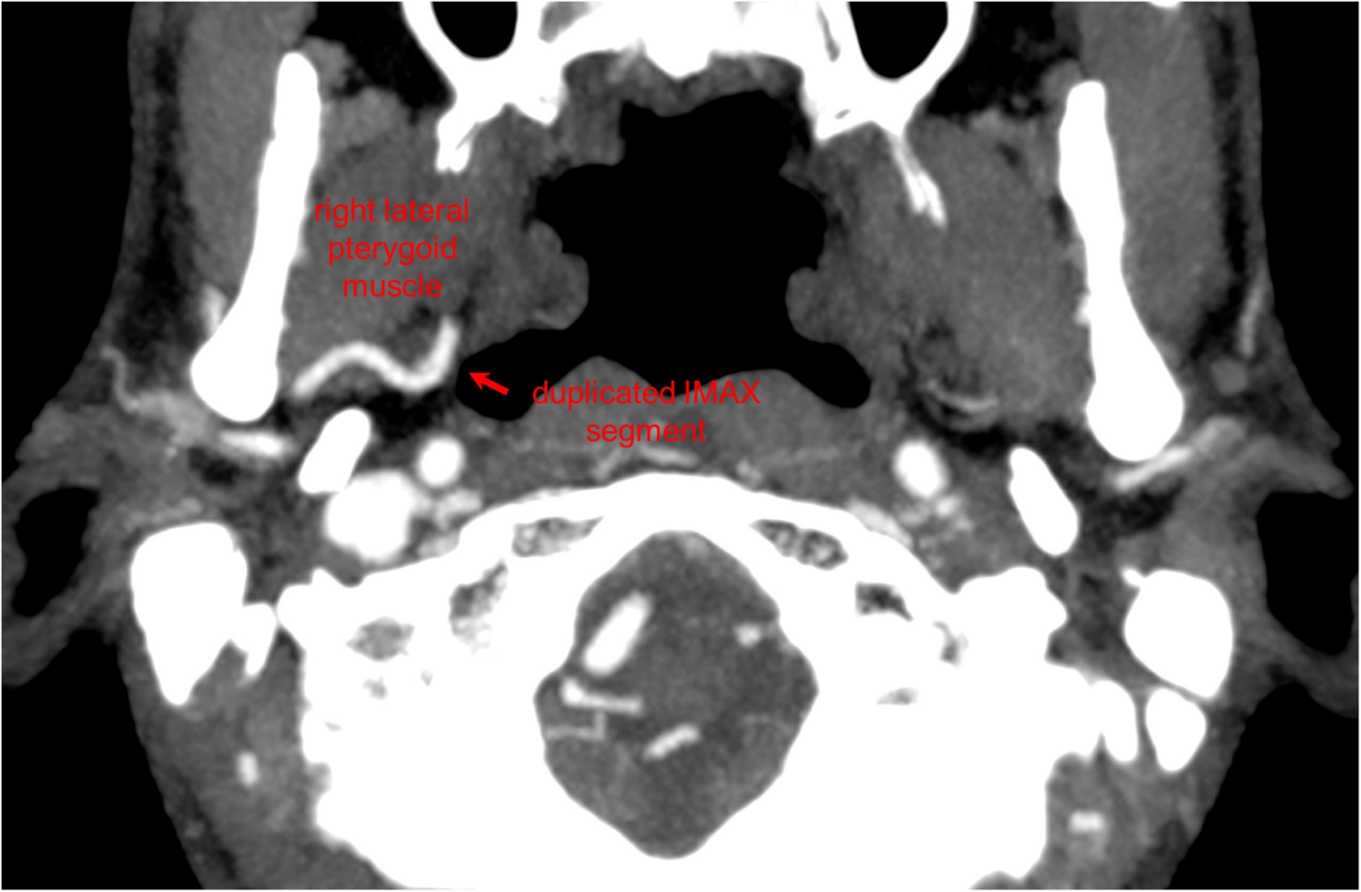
Axial maximum intensity projection (MIP) CT image demonstrates a deep course of the duplicated right internal maxillary artery (IMAX) segment relative to the right lateral pterygoid muscle.
